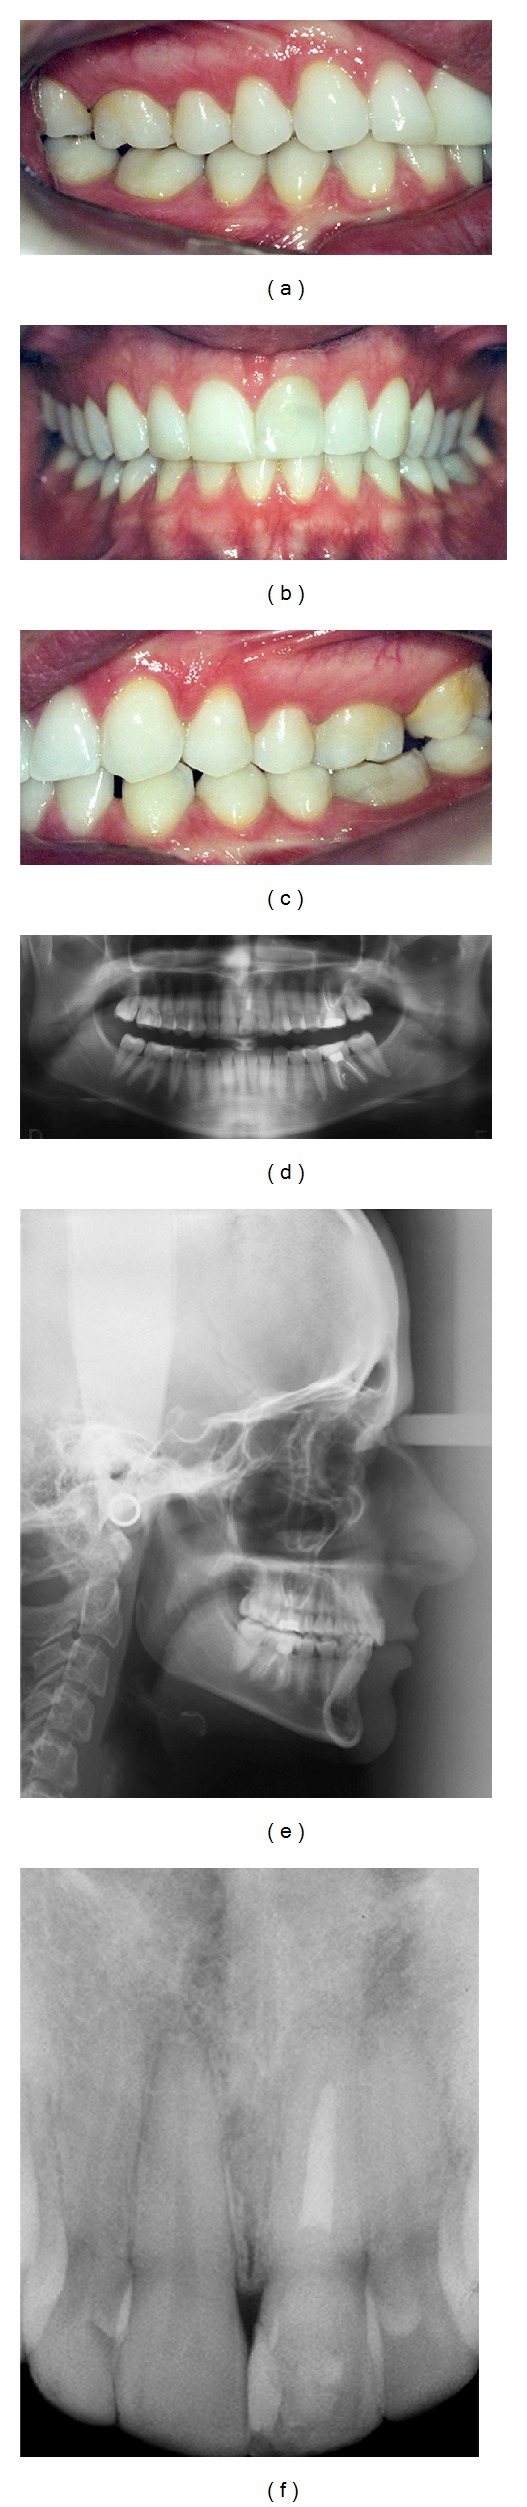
(a, b, c) Preoperative views; (d) panoramic radiography; (e) cephalometric radiography; (f) periapical radiography showing no significant changes to the maxillary left central incisor.
